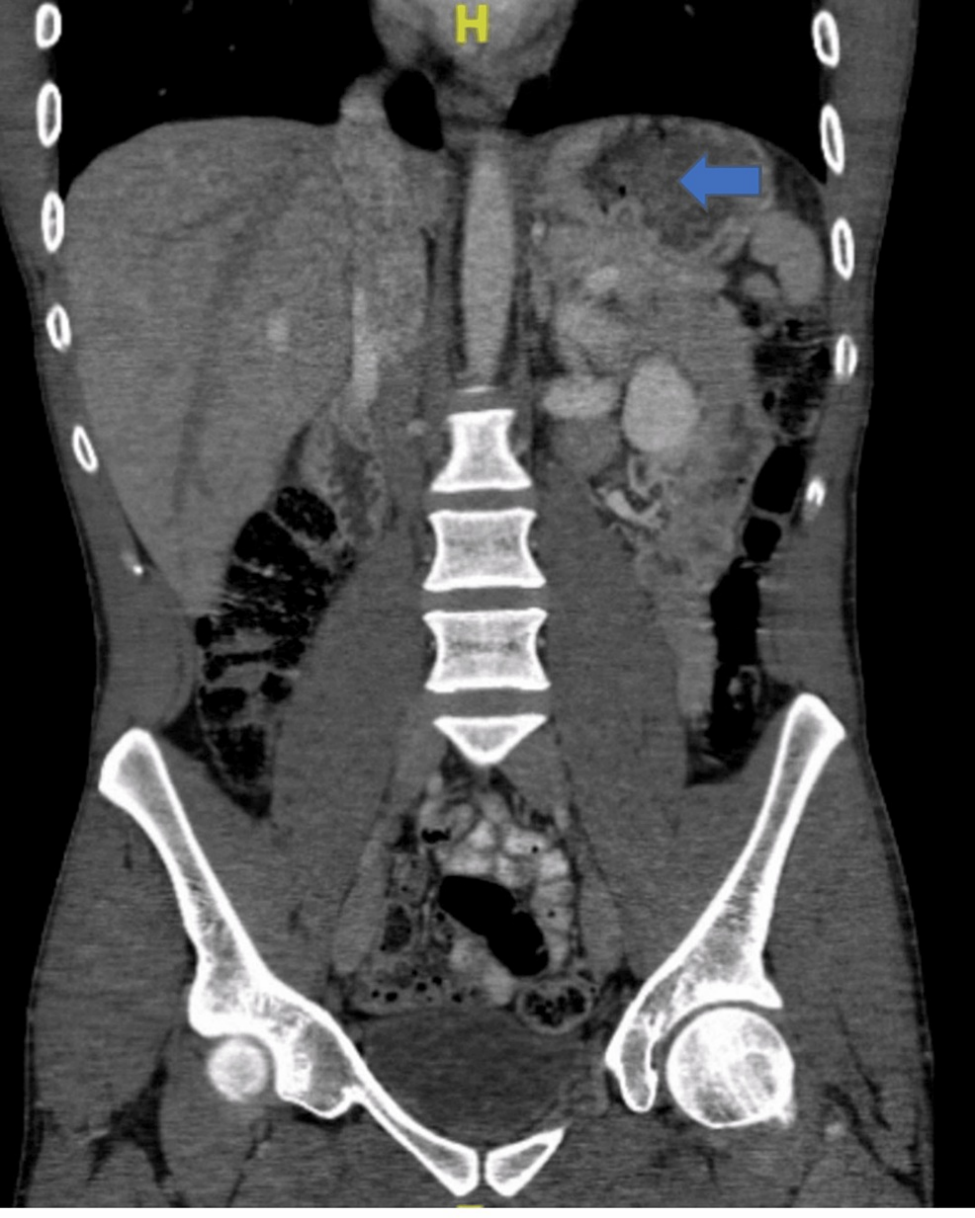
Coronal view of CT abdomen and pelvis without contrast Circumferential thickening of the gastric wall with multiple small collections of air within the gastric wall and mild peri-gastric fat stranding (blue arrow points to intramural gas in the stomach).
